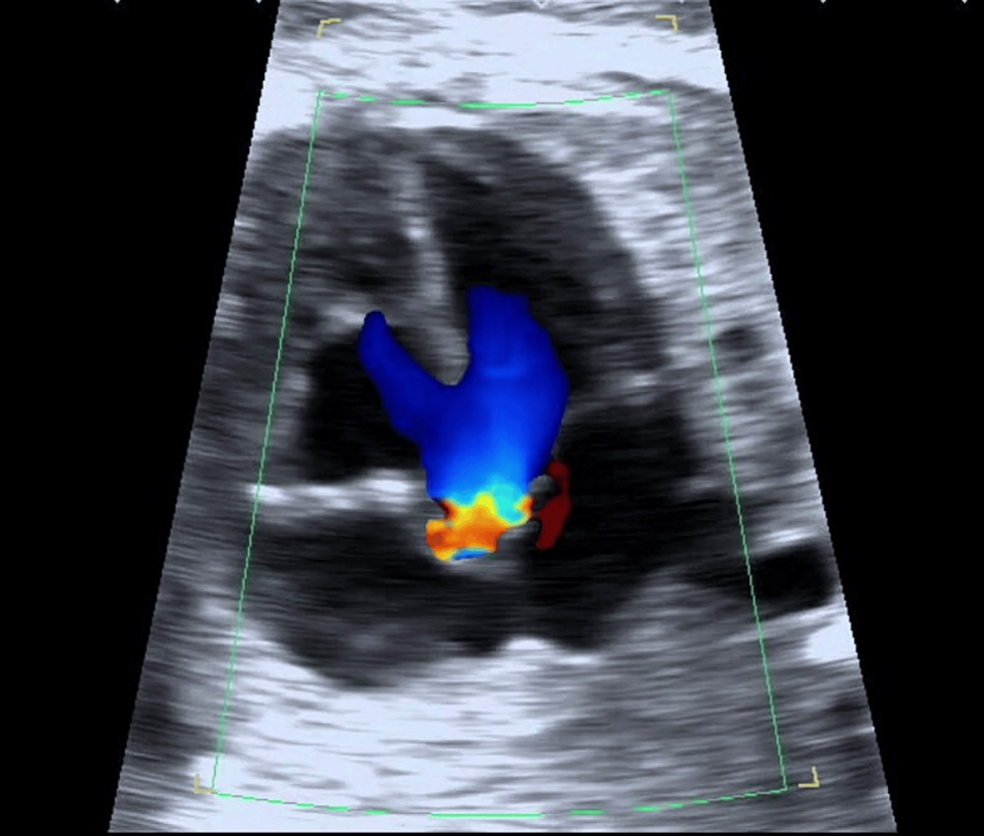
Echocardiogram suggesting overriding of the aorta
